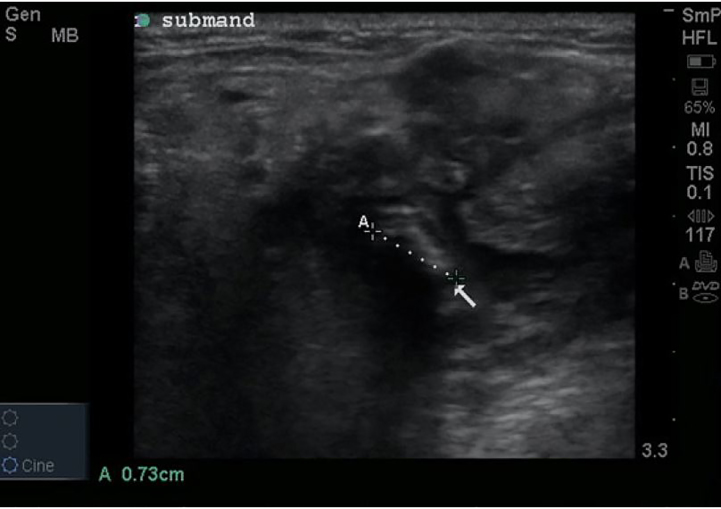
Ultrasound image of a 7.3 mm sialolith obstructing the salivary duct (arrow). Computed tomography confirmed an eight mm stone within the right Wharton’s duct (Image 3).
